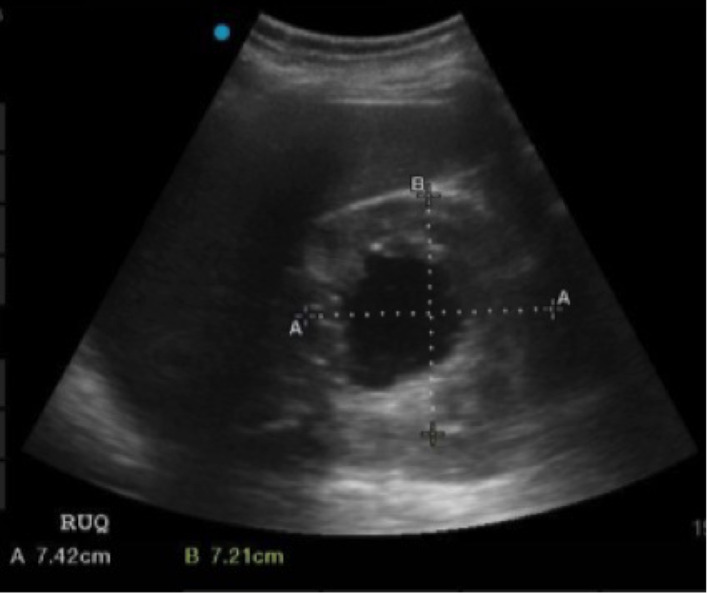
Right upper quadrant mass seen on emergency physician-performed point-of-care ultrasound. Measurements showing 7.42 × 7.21 cm in cephalad-caudal and lateral dimensions, respectively.
